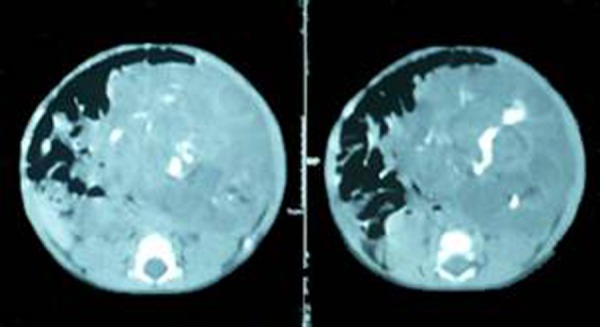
Figure 1: CT scan showing solid and cystic components with internal calcifications.
